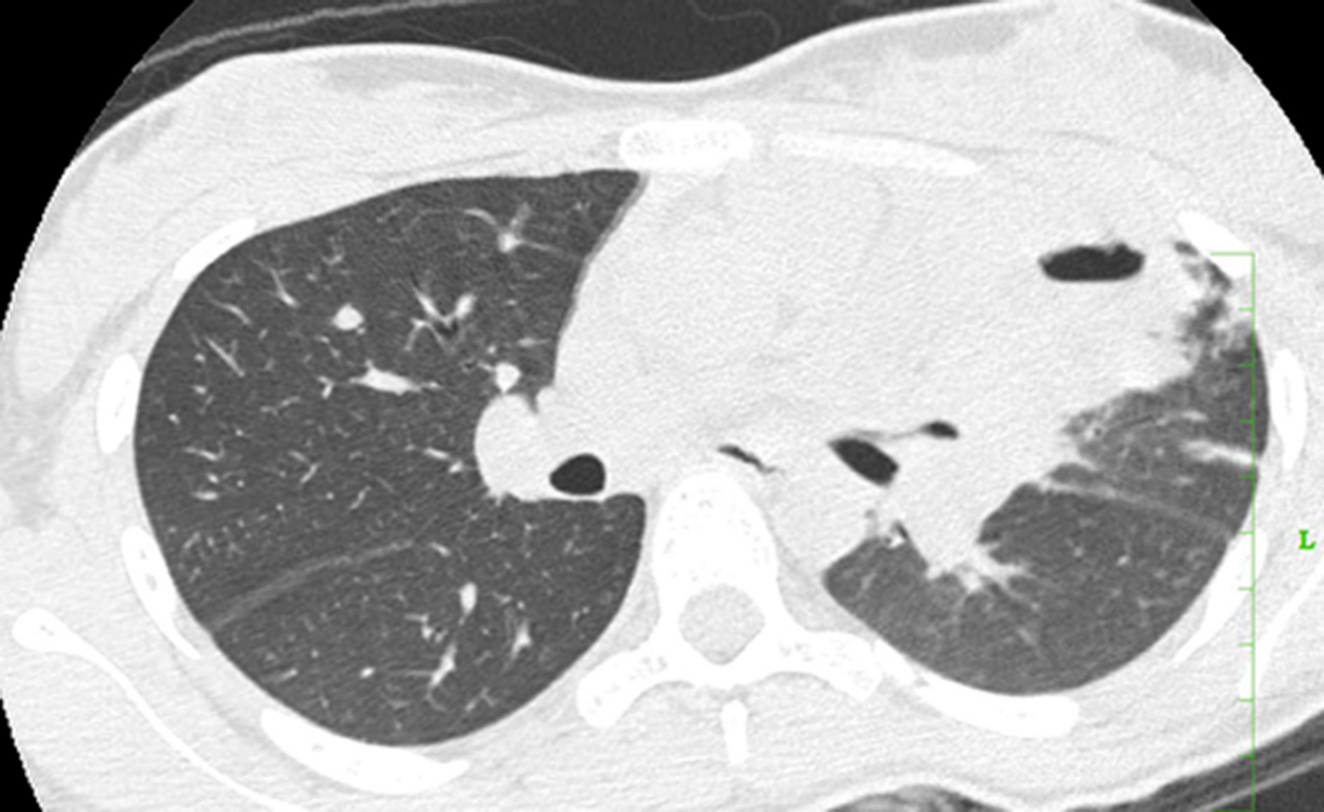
Case 2(c): Axial CT image of the chest in lung window shows cavitating consolidation in the left upper lobe.
